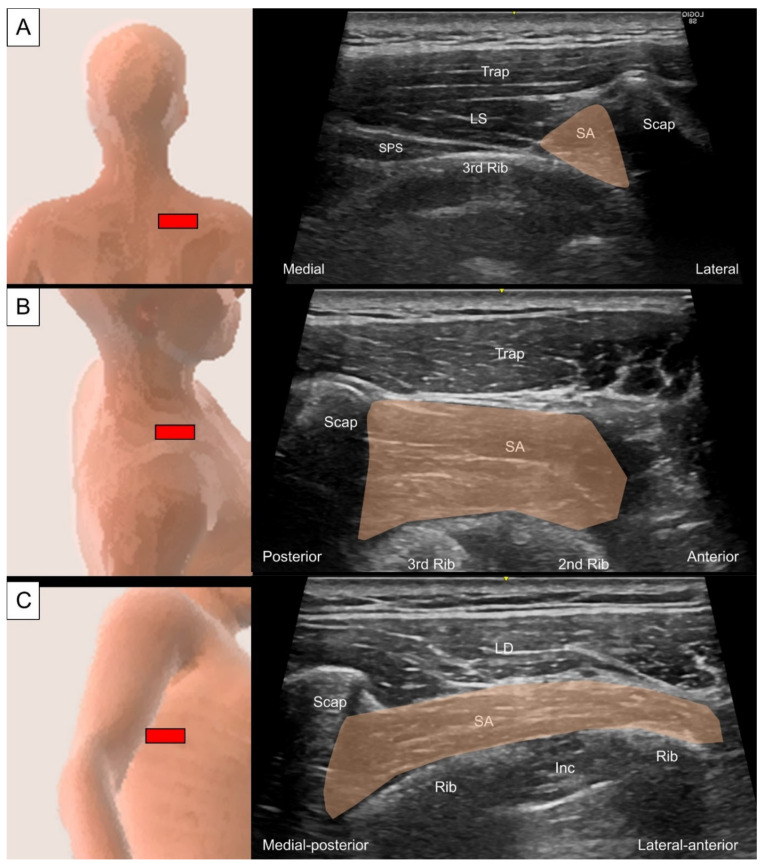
Ultrasound imaging of the serratus anterior (SA, skin color shade) (A) lying underneath the scapula, (B) crossing the intercostal spaces to insert on the ribs, and (C) running at the lateral trunk region. Trap, trapezius; Scap, scapula; LS, levator scapulae; SPS, serratus posterior superior; LD, latissimus dorsi; Inc, intercostal muscle.
